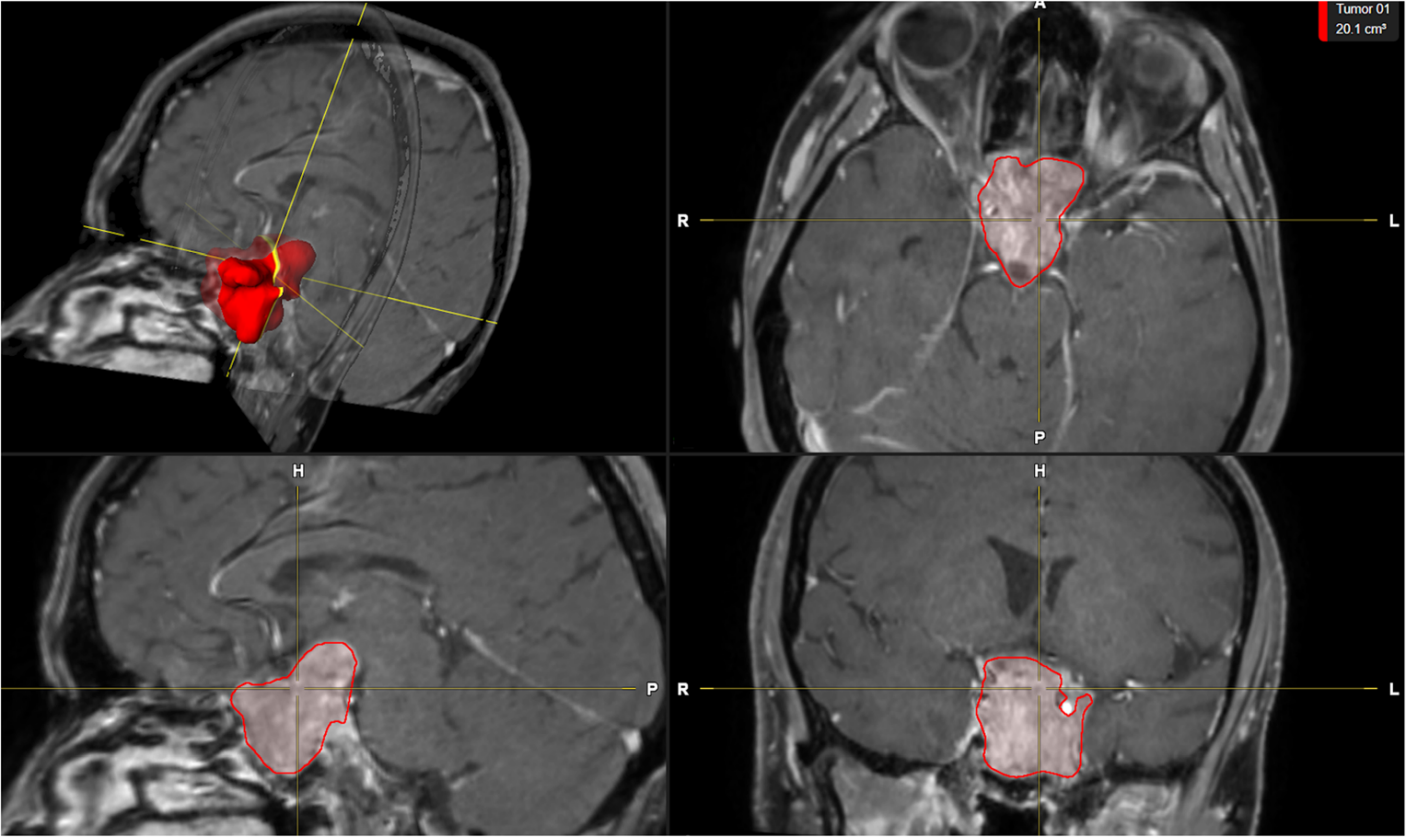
Volumetric assessment of tumor size (red), cavernous sinus invasion (in this case Knosp grade 4), and tumor invasiveness through the sella floor (Hardy classification, in this case classified as class 3) using the Brainlab software on preoperative MRI
